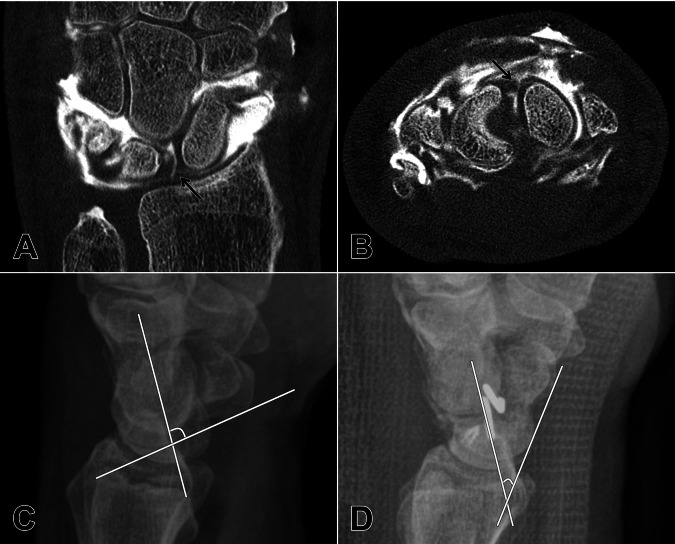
Suffering a motorcycle accident, a 62-year-old man was admitted to the emergency department for increasing left wrist pain and swelling. Cone-beam CT arthrography revealed a destabilizing tear of the scapholunate ligament’s dorsal segment (A/B, *black arrows*), while the previously taken radiograms displayed a widened scapholunate angle indicative of dorsal intercalated segment instability (C). Without patient repositioning between contrast injection into the radiocarpal compartment under fluoroscopic guidance and the completion of 3D imaging, the entire procedure required less than 5 min time. Subsequently, the patient underwent ligament suture as well as scapholunate and scaphocapitate transfixation to re-establish the stability of the proximal carpal row. Surgical success is indicated by marked narrowing of the scapholunate angle in the postoperative lateral radiogram (D)
